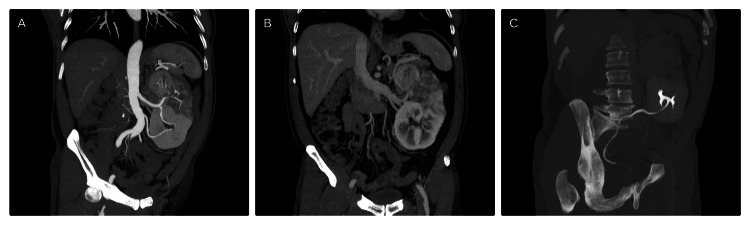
Renal cell carcinoma in crossed fused renal ectopia Coronal contrast-enhanced CT under the maximum intensity projection in arterial phase (A), corticomedullary renal phase (B), and delayed excretory renal phase (C). The arterial phase (A) shows a large unilateral kidney on the left side, with hypoenhancing mass in the upper pole. Two renal arteries are emerging from the abdominal aorta. The corticomedullary renal phase (B) shows a single draining renal vein into the IVC, with the absence of a contralateral vein. The delayed excretory renal phase (C) shows the lower moiety ureter crossing the midline, with a conventional bladder insertion. No contrast is seen within the upper moiety ureter.
